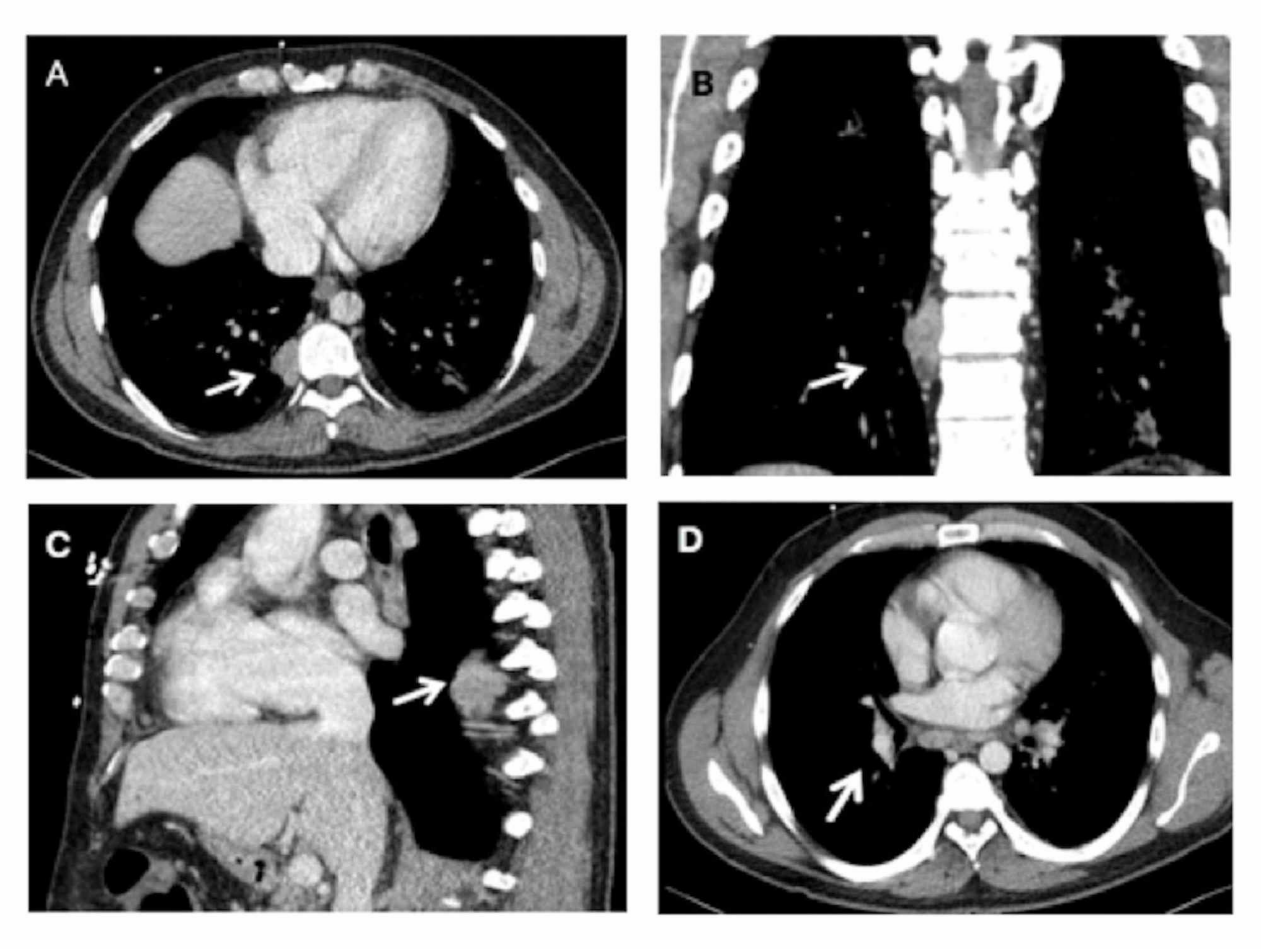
(A) Axial, (B) coronal, and (C) sagittal view of the right paravertebral posterior mediastinal mass or lymphadenopathy. (D) Diffuse mediastinal lymphadenopathy on the chest CT scan
